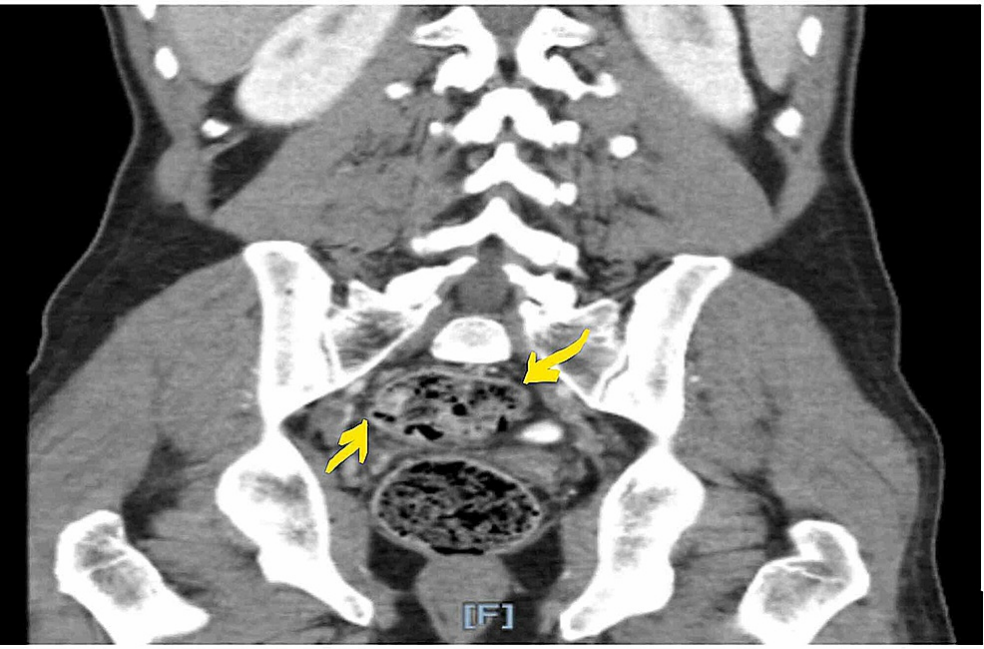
CT abdomen and pelvis CT image in the coronal plane showing a multilobular solid and cystic lobular mass in the right pelvis measuring 5.9 x 4.4 x 5.6 cm (yellow arrows), abutting the posterior dome of the bladder CT: computed tomography
